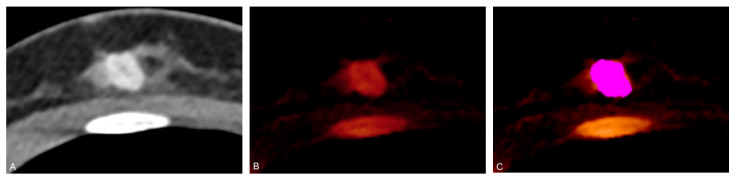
Axial contrast-enhanced dual-energy CT (DECT) scan of a 47-year-old woman with grade 1 luminal A invasive ductal carcinoma in the right breast. Linearly blended M_0.6 series (A) demonstrate a contrast-enhancing mass; pure iodine maps (B) reconstructed from DECT datasets display iodine content within the lesion. A three-dimensional volume of interest (VOI) (C) is placed semi-automatically on the iodine map for radiomic analysis.
